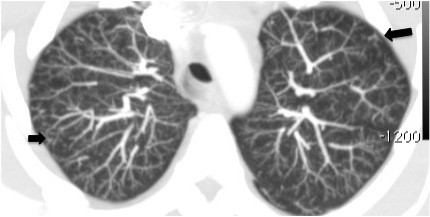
CT axial series of the upper lobes with maximum intensity projection showing diffuse centrilobular nodular opacities with ground-glass attenuation associated with tree-in-bud pattern.
